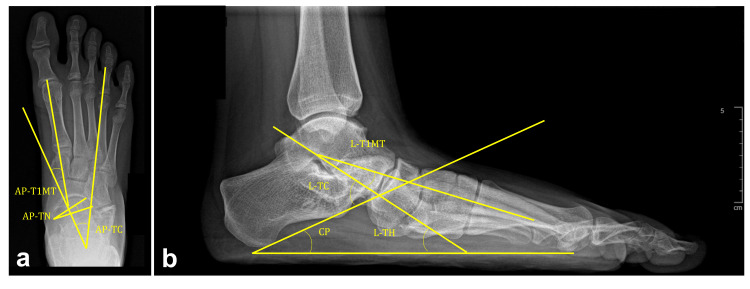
Anteroposterior and lateral weight-bearing radiographs a: Radiography revealing the AP-T1MT, AP-TC, and AP-TN coverage angle; b: Radiography showing the L-T1MT, L-TC, L-TH, and CP angle AP-T1MT: Anteroposterior talo-first metatarsal, AP-TC: Antero-posterior talocalcaneal, AP-TN: Anteroposterior talonavicular, L-T1MT: Lateral talo-first metatarsal, L-TC: Lateral talocalcaneal, L-TH: Lateral talohorizontal, CP: Calcaneal pitch
